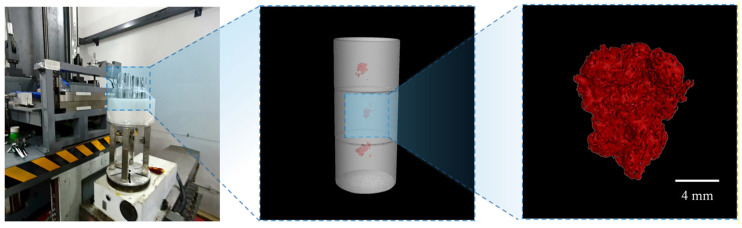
3D morphology of defects characterized by high-energy CT.
